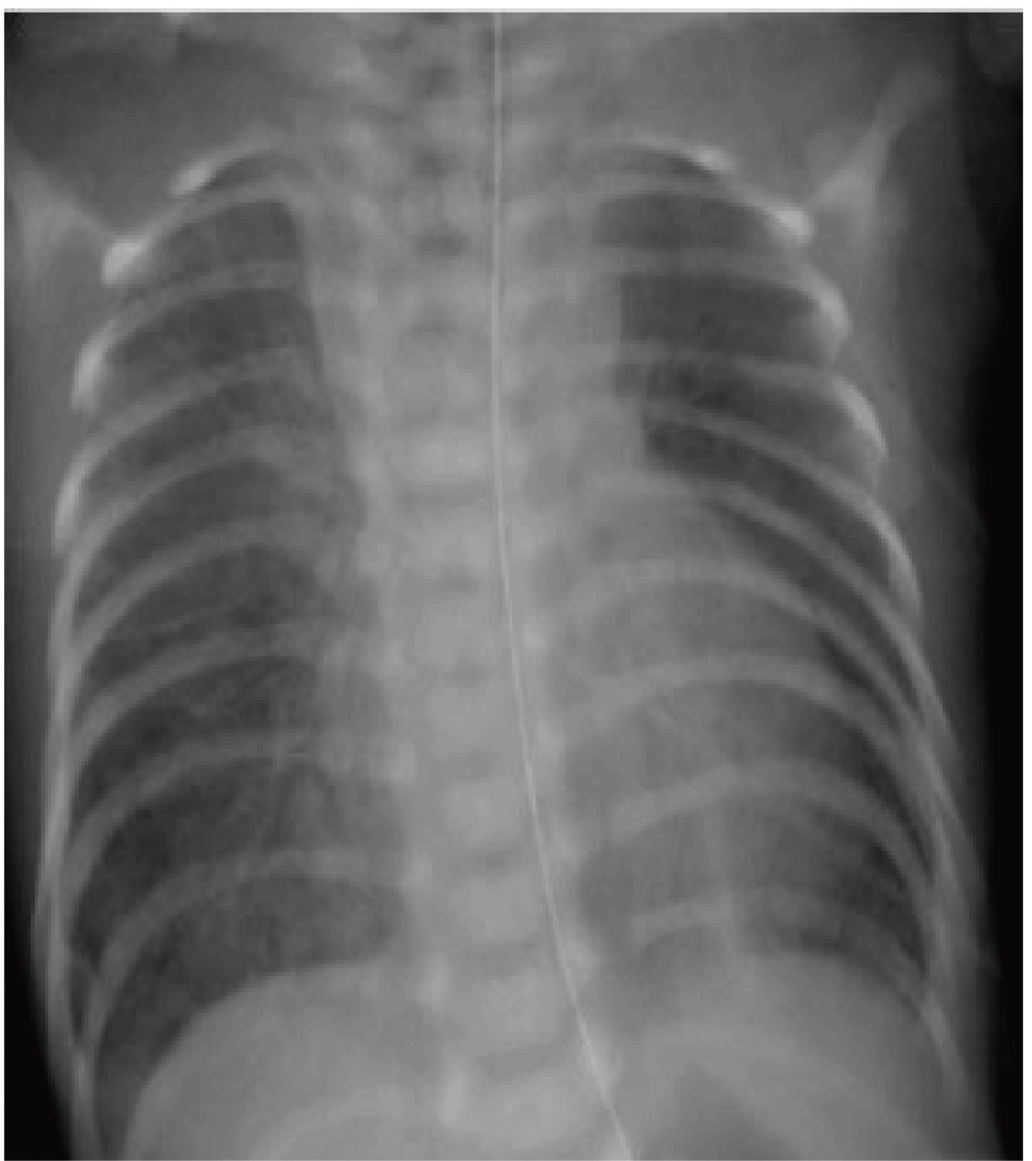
Postnatal chest radiography.
